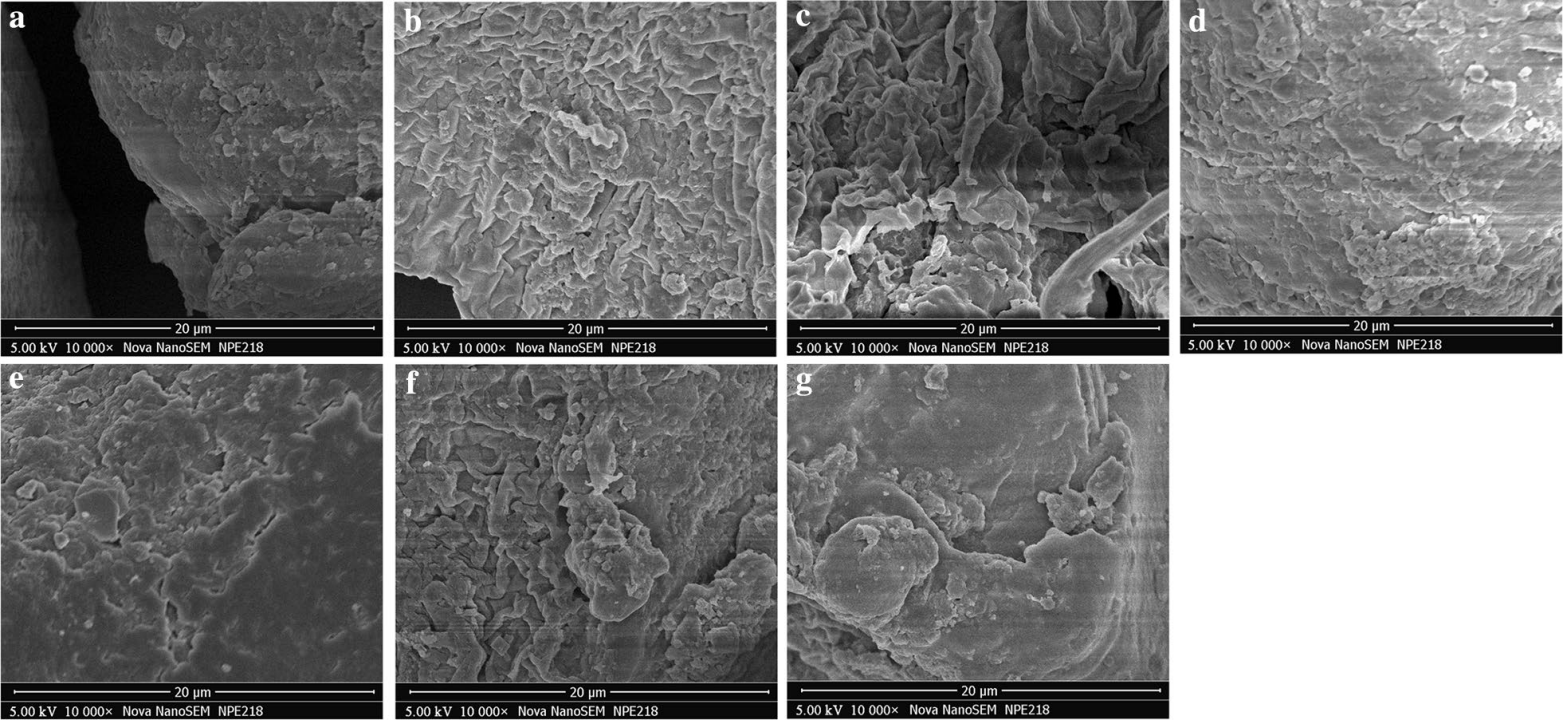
FESEM images of raw and treated materials under different extraction conditions. a Raw materials; b Ultrasonic extraction 8 min; c Ultrasonic extraction 20 min; d SD extraction 20 min; e Maceration extraction 20 min; f SD extraction 1 h and g Maceration extraction 2 h
